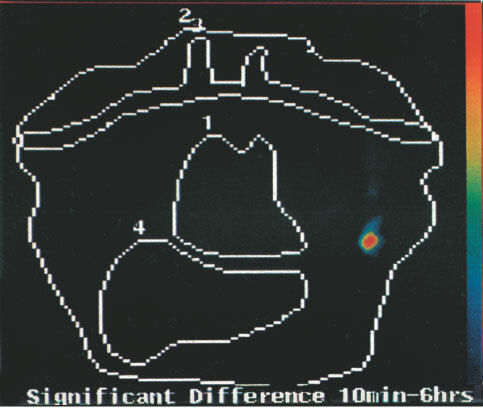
Patient CT 5, Tc-99m hHMFG-1 radioimmunoscintigraphy. Negative left axilla nodes and positive breast cancer.
